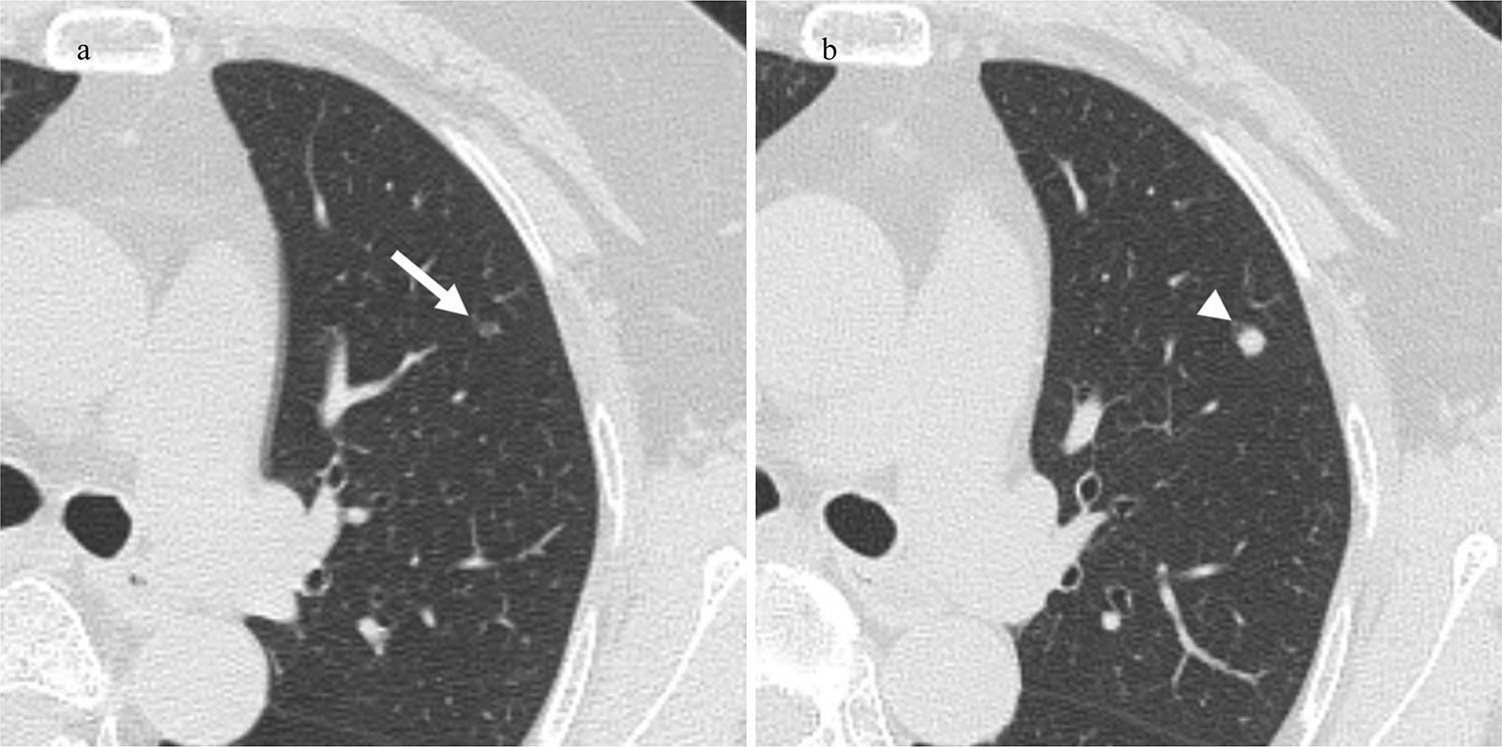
A 72-year-old woman with cutaneous melanoma. a. CT after 5 years of operation shows a 3 mm-sized GGN in the left upper lobe (white arrow). b. CT obtained 15 months after Fig. 4a shows a GGN that has grown to 5 mm and turned to a solid nodule (white arrowhead). Surgery was performed, and the nodule was diagnosed as a metastasis from malignant melanoma
